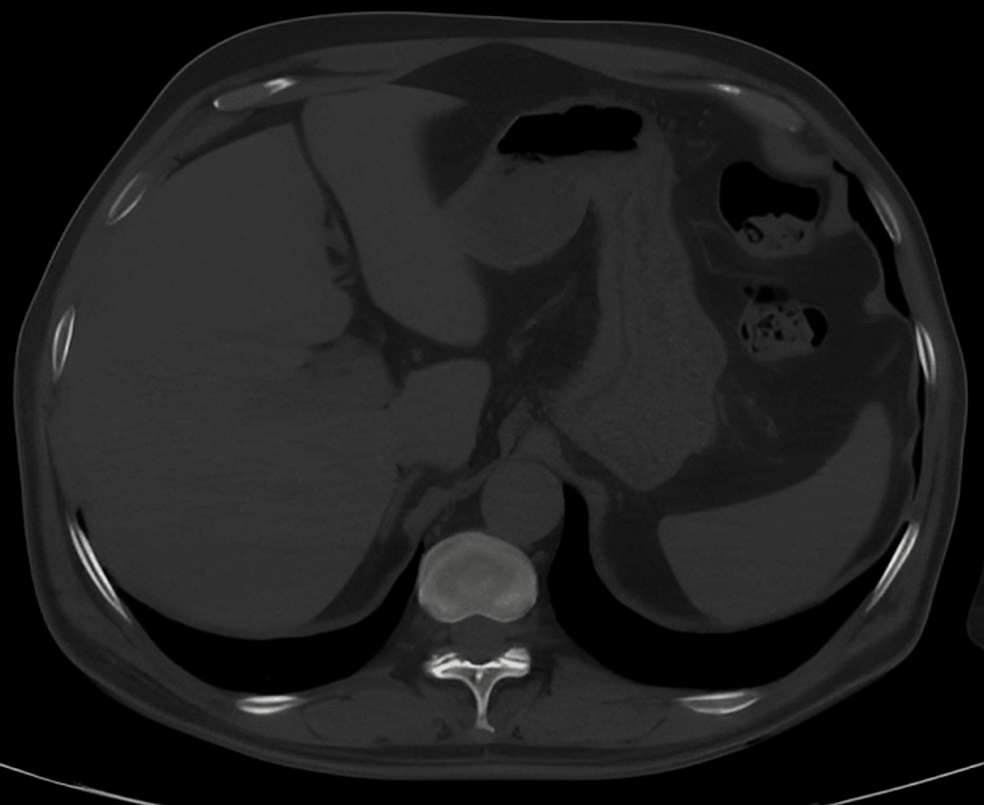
CT - abdomen CT screening of the abdomen axial section showed no discrete lesions in the liver and spleen CT: computed tomography
